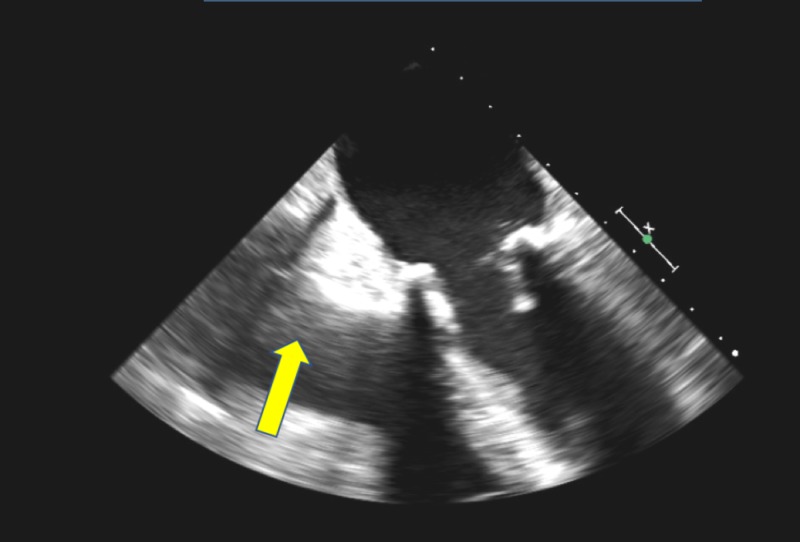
Post-surgery transesophageal echocardiogram (TEE) showing expansion of the right atrium Post-surgery TEE showing expansion and improved filling of the right atrium (yellow arrow) following surgical evacuation of the pericardial hematoma
